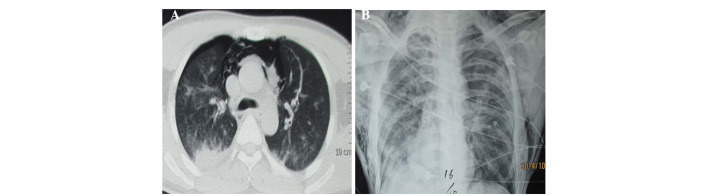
(A) Chest computed tomography scan image of the patient on day 1. The scan shows mediastinal emphysema and pneumothorax. (B) Chest X-ray image of the patient on day 2 following ventilation in the intensive care unit. The image shows pneumothorax and subcutaneous emphysema.
